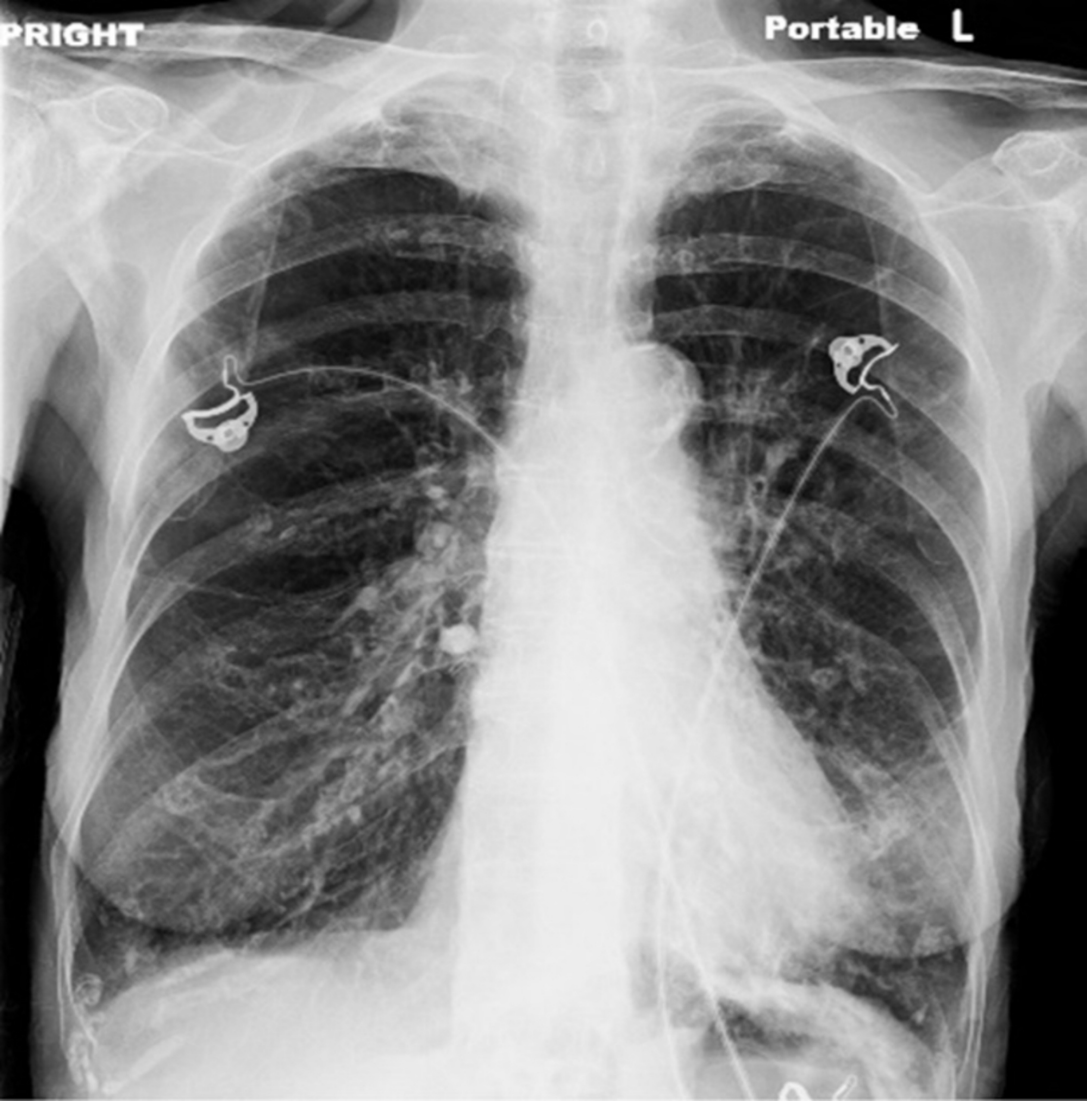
Chest X-ray revealing hyper-inflated lungs without any focal consolidation.
